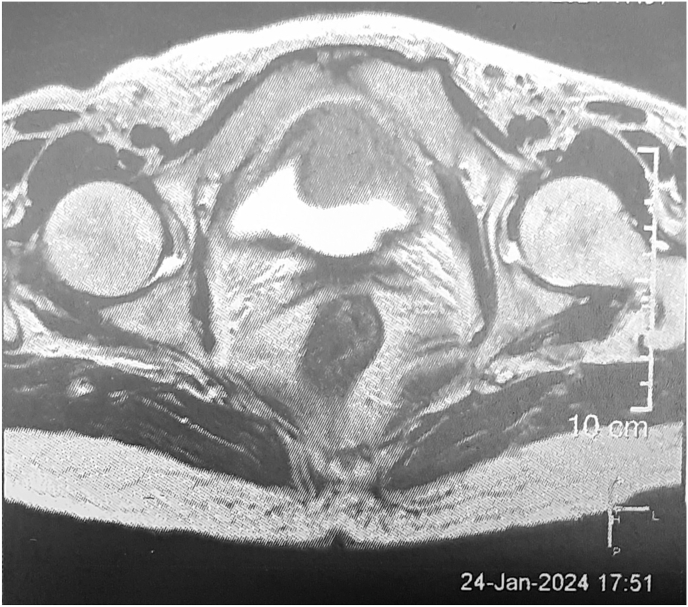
Magnetic resonance imaging of bladder mass Bladder mass was detected on the anterior wall of the urinary bladder with obvious enhancement and hyperintensity on T2-weighted imaging.
